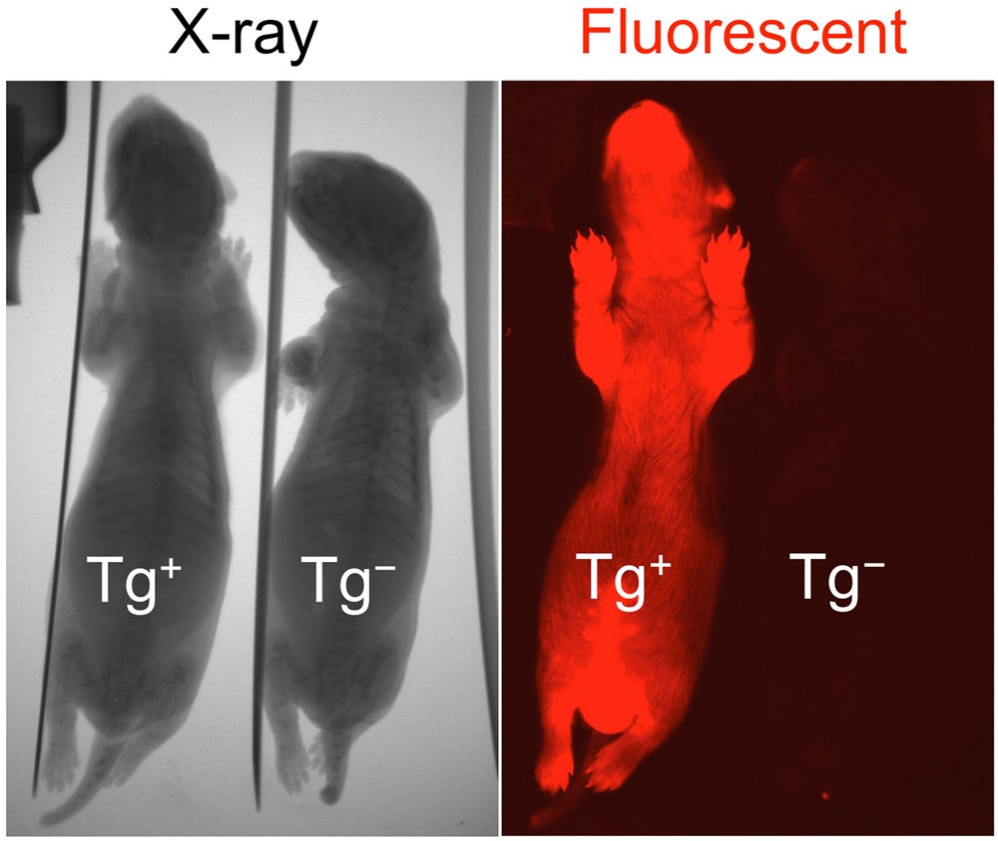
Whole body tdTomato expression in a transgenic ferret. tdTomato transgene expression is shown for a juvenile F1 transgene positive ferret founder A1 (Tg^+^) and transgene negative littermate (Tg^−^). X-ray images are shown in the left panel and fluorescent images are shown in the right panel.
